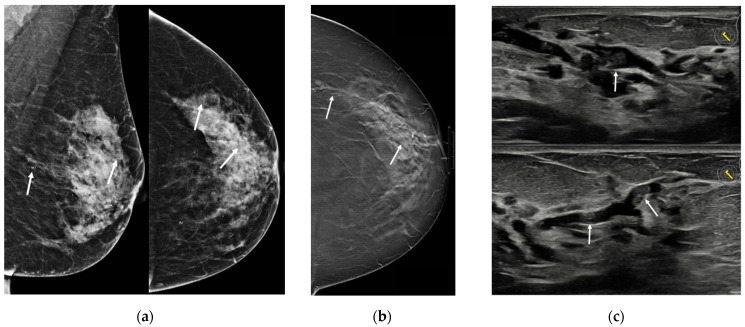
A 55-year-old woman presenting with unilateral bloody nipple discharge. (a) Mammography in medio-lateral view and cranio-caudal view showed multiple scattered calcifications (arrows). (b) Tomosynthesis slice in the CC view showed multiple dilated ducts from the retroareolar region to the outer quadrants (arrows). (c) Ultrasound demonstrated multiple dilated ducts partially filled with intraluminal content (arrows).
